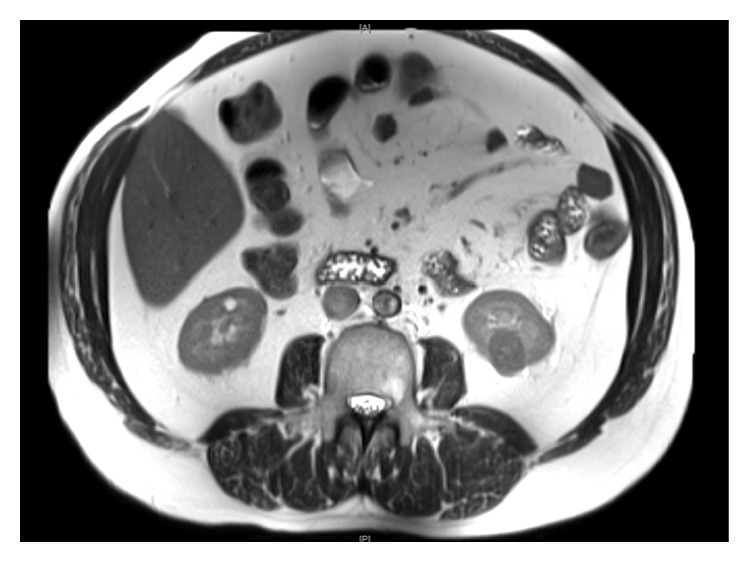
Magnetic resonance imaging (MRI) showing a 26 × 21 mm left renal lower pole mass with a low T2 signal.
